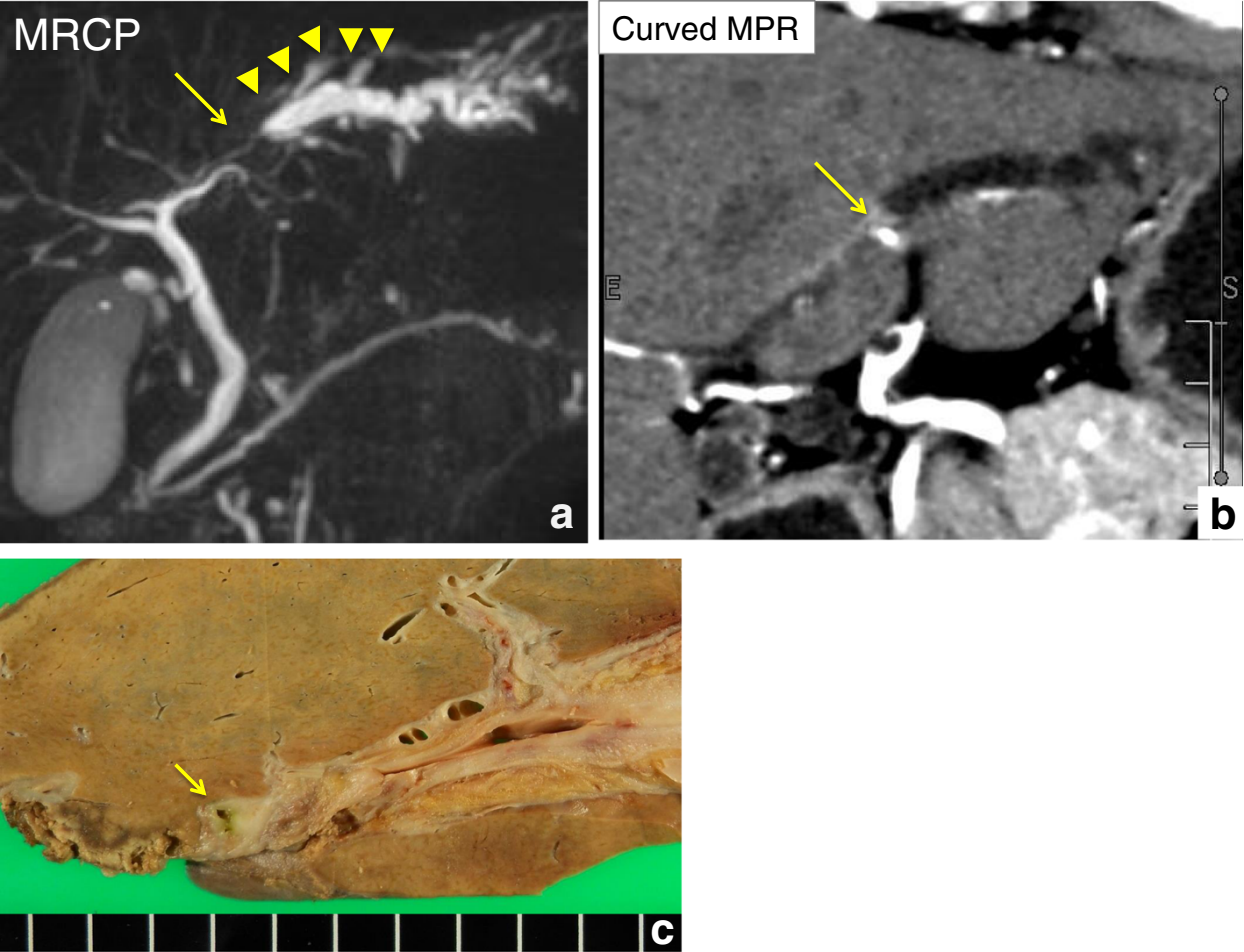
Radiological and macroscopic features of the bile duct and liver tumor. a Magnetic resonance cholangiopancreatography (MRCP). The B2 bile duct is obstructed (arrow) and dilated at its periphery (arrow heads). b Curved multiplanar reconstruction image of contrast-enhanced CT. The liver tumor is visualized as a high-intensity area in the arterial phase (arrow). c Macroscopic view of the tumor. Grayish tumor tissue is observed (arrow)
